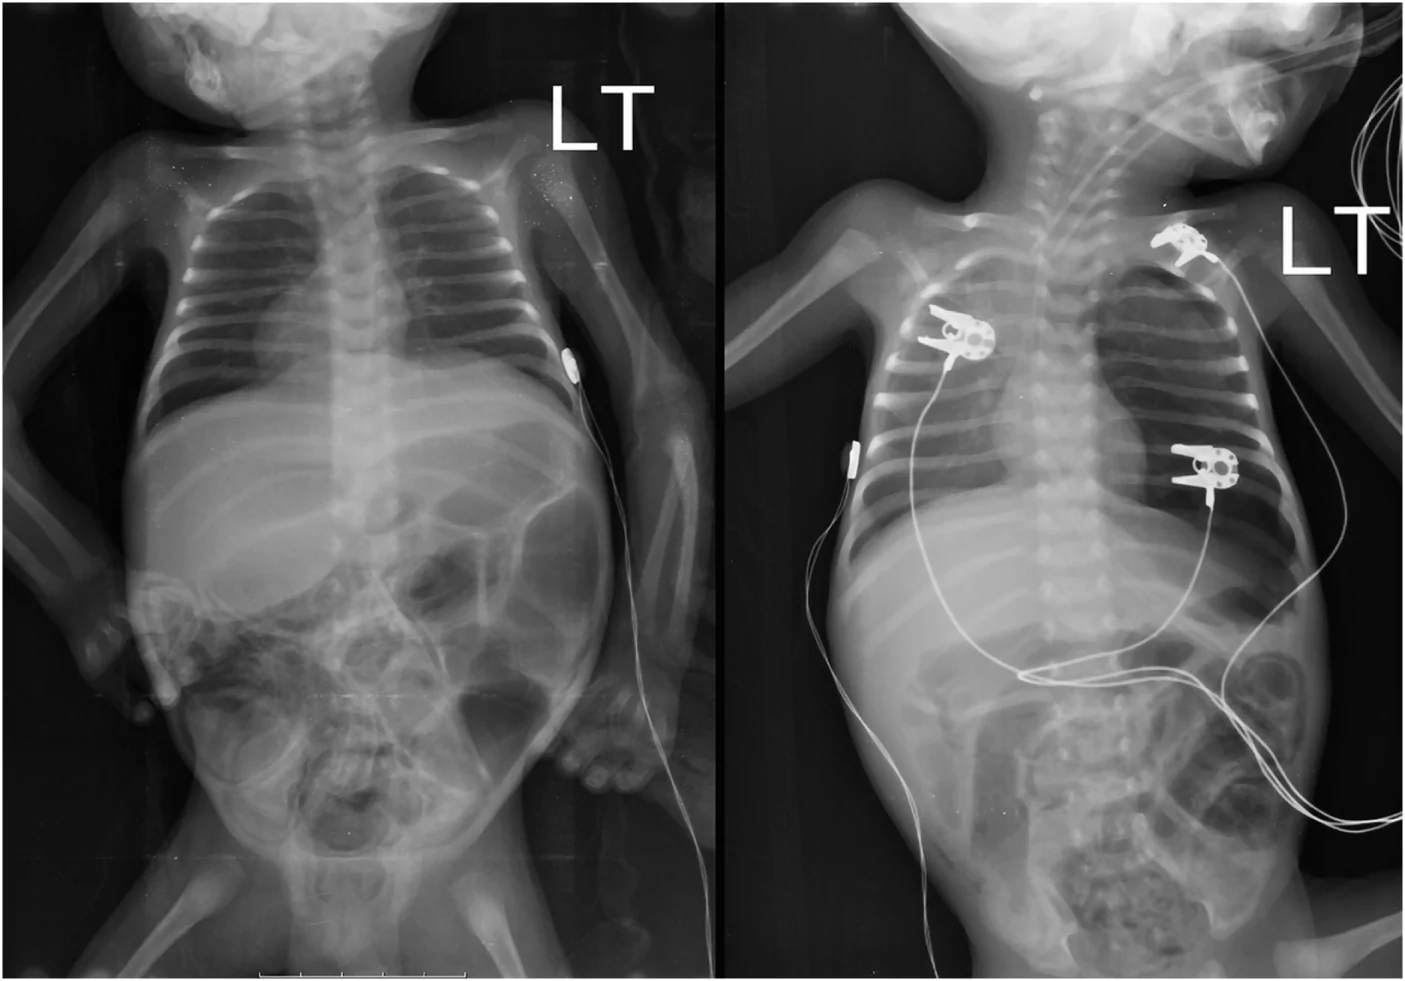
An abdominal radiograph shows pneumatosis intestinalis consistent with necrotizing enterocolitis (Bell stage II) during the sixth postoperative week.
